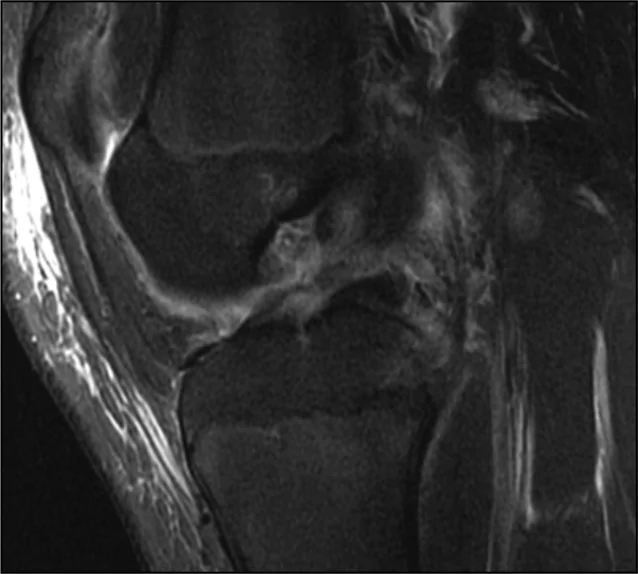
Sagittal MRI demonstrating midsubstance rupture of the ACL.
